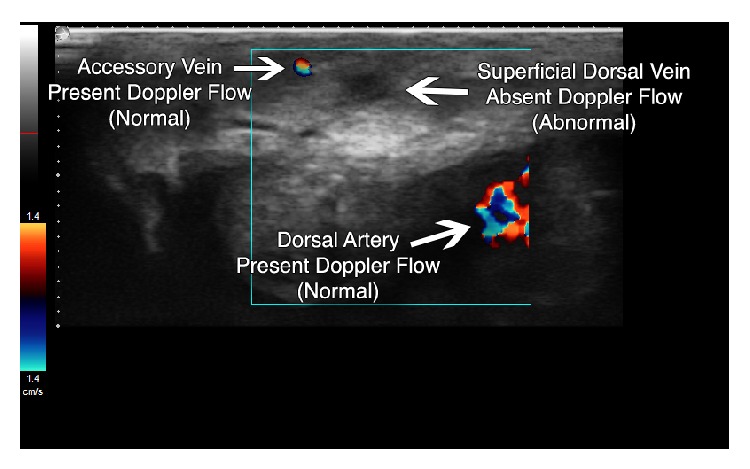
Color Doppler ultrasonography showing absent flow in right superficial vein when compared to accessory vein and dorsal artery, which both demonstrate normal flow (depth set at 2 cm).
